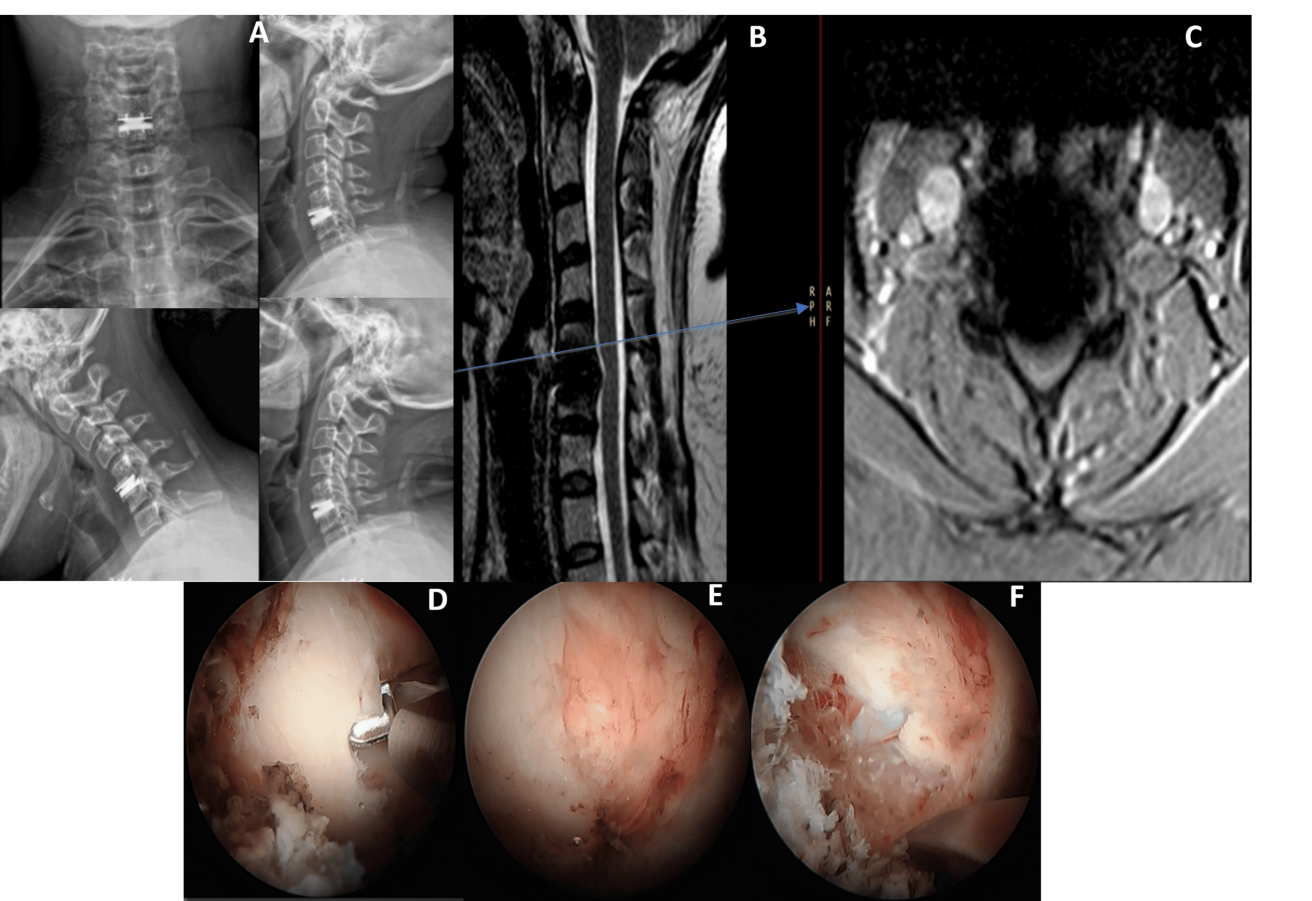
Cervical spine revision surgery in Case 1. (A) Preoperative anteroposterior, lateral, and dynamic cervical X-rays, status post C5/C6 TDR, showing TDR displacement with no postoperative improvement and persistent brachalgia. (B) Axial and sagittal T2-weighted cervical MRI demonstrating artifacts from the TDR, correlating with her persistent symptoms. (C) Intraoperative image following right posterior cervical C5/C6 decompression and discectomy, showing a laminotomy performed after initial TDR failed to alleviate symptoms. (D) Intraoperative image depicting the V point between the C5 and C6 lamina after decompression surgery. (E) Intraoperative image showing the decompressed nerve root with bleeding controlled using Surgicel (Ethicon, Inc., Raritan, New Jersey, United States) following posterior cervical decompression and discectomy. TDR: total disc replacement
